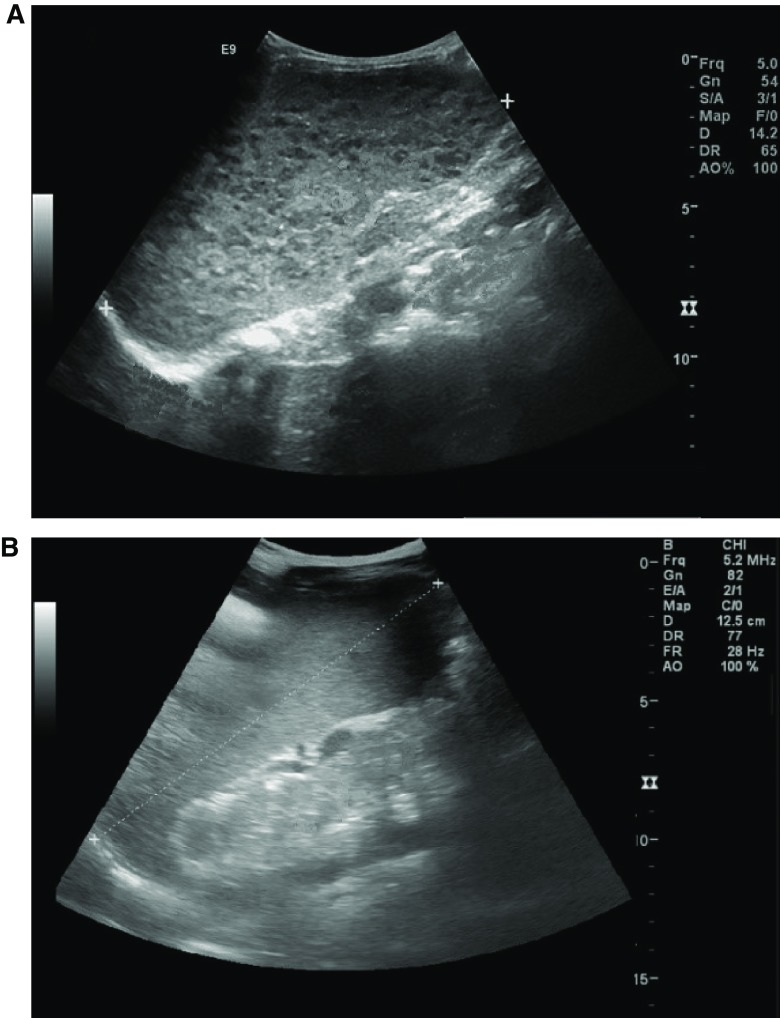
Ultrasound scan of enlarged spleen in (
